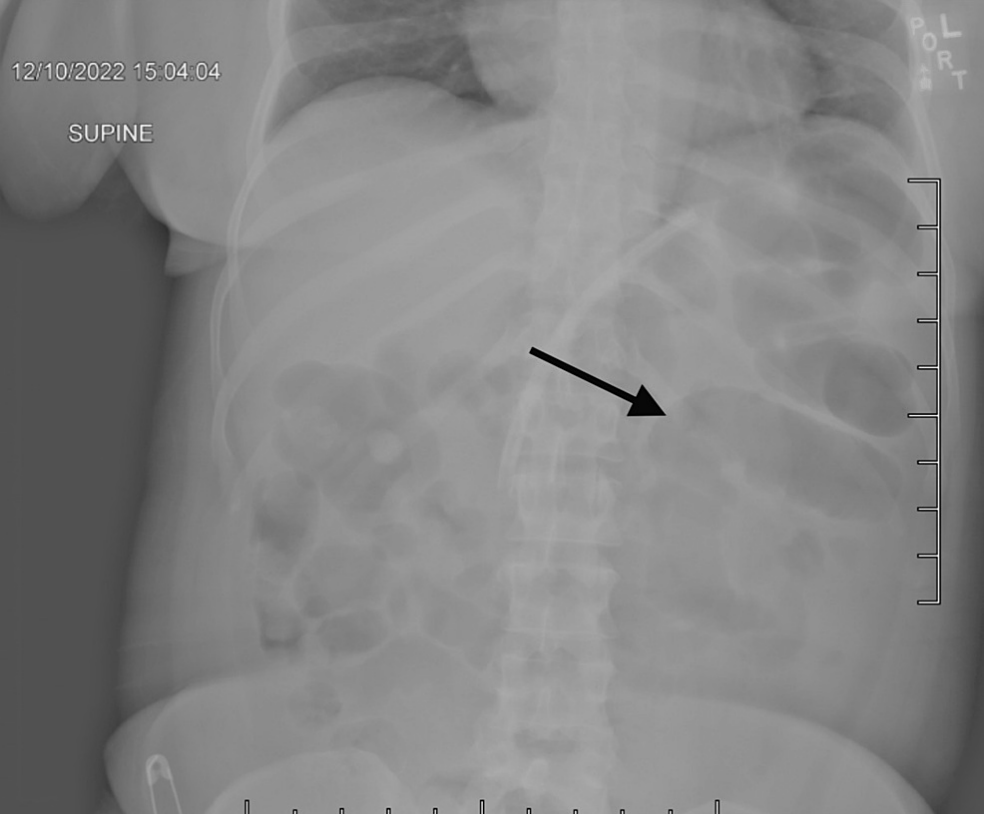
Abdominal X-ray with an arrow pointing to the dilated bowel loops
